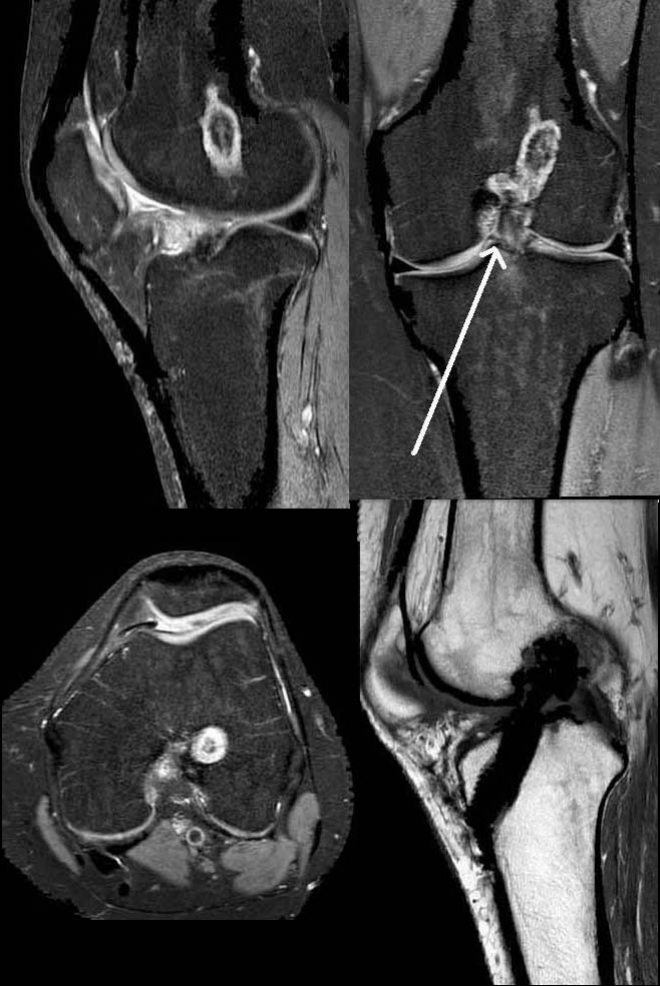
The MRI (PDW FS sagittal, PDW FS coronal, PDW FS axial, T1 TSE sagittal) showed an intact continuity of the ACL graft and a possible tibial cyclops lesion.
